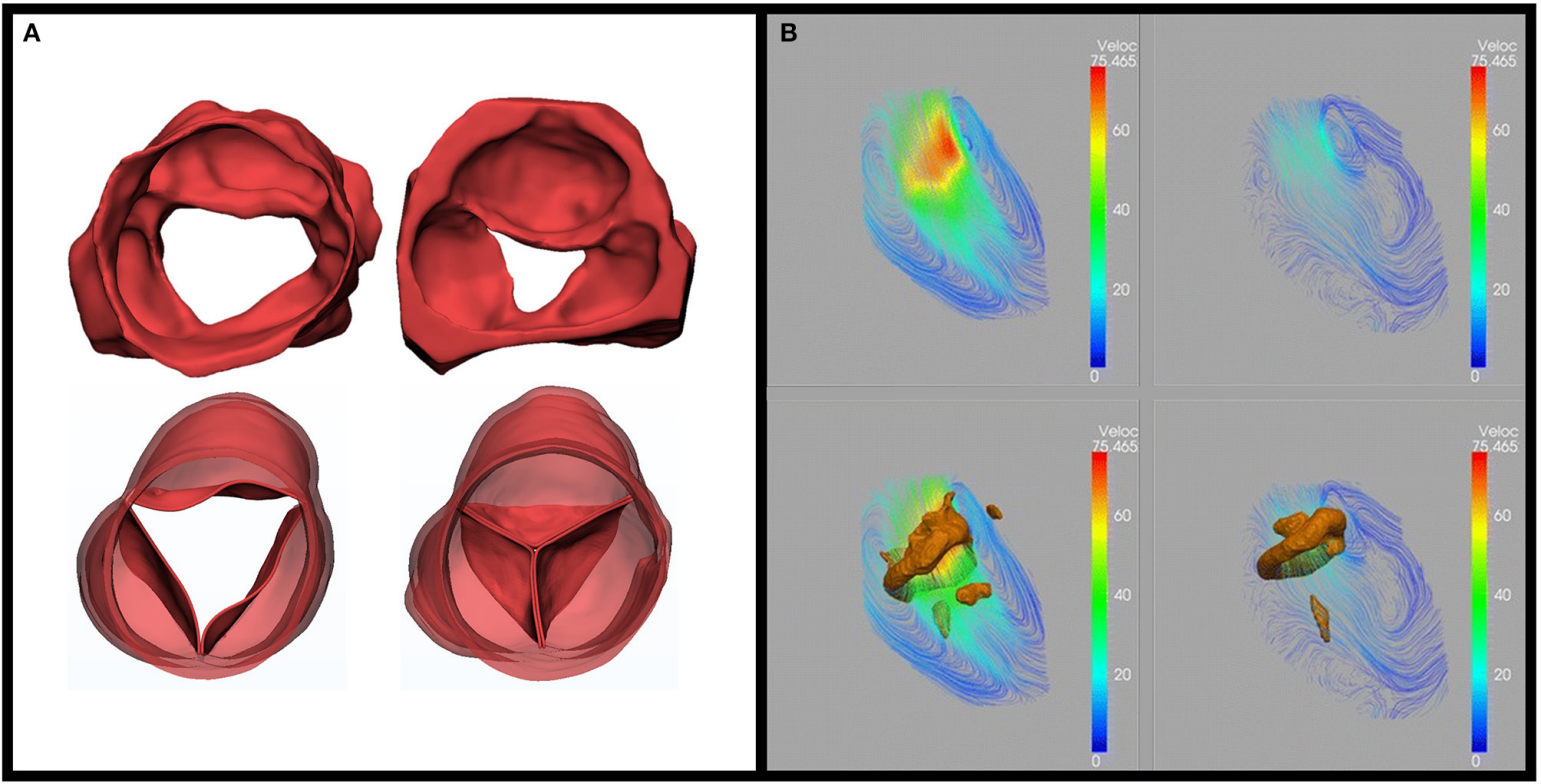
Role of multimodality imaging. (A) Three-dimensional 3D segmentation from computed tomography for an advanced rheumatic aortic valve unsuitable for repair (top) and after replacement (Ross operation) (bottom) in systole (left) and diastole (right). The images show shrunken and motion restricted valve leaflets preoperatively, no regurge, and excellent coaptation postoperatively adapted from Afifi et al. (15). (B) Use of MRI 4D flow: streamline and vortex rings. On a cross-sectional view of LV during (a) early filling and (b) late filling from Elbaz, et al. Vortex flow during early and late left ventricular filling in normal subjects: Quantitative characterization using retrospectively-gated 4D flow cardiovascular magnetic resonance and three-dimensional vortex core analysis. Journal of cardiovascular magnetic resonance (20).
